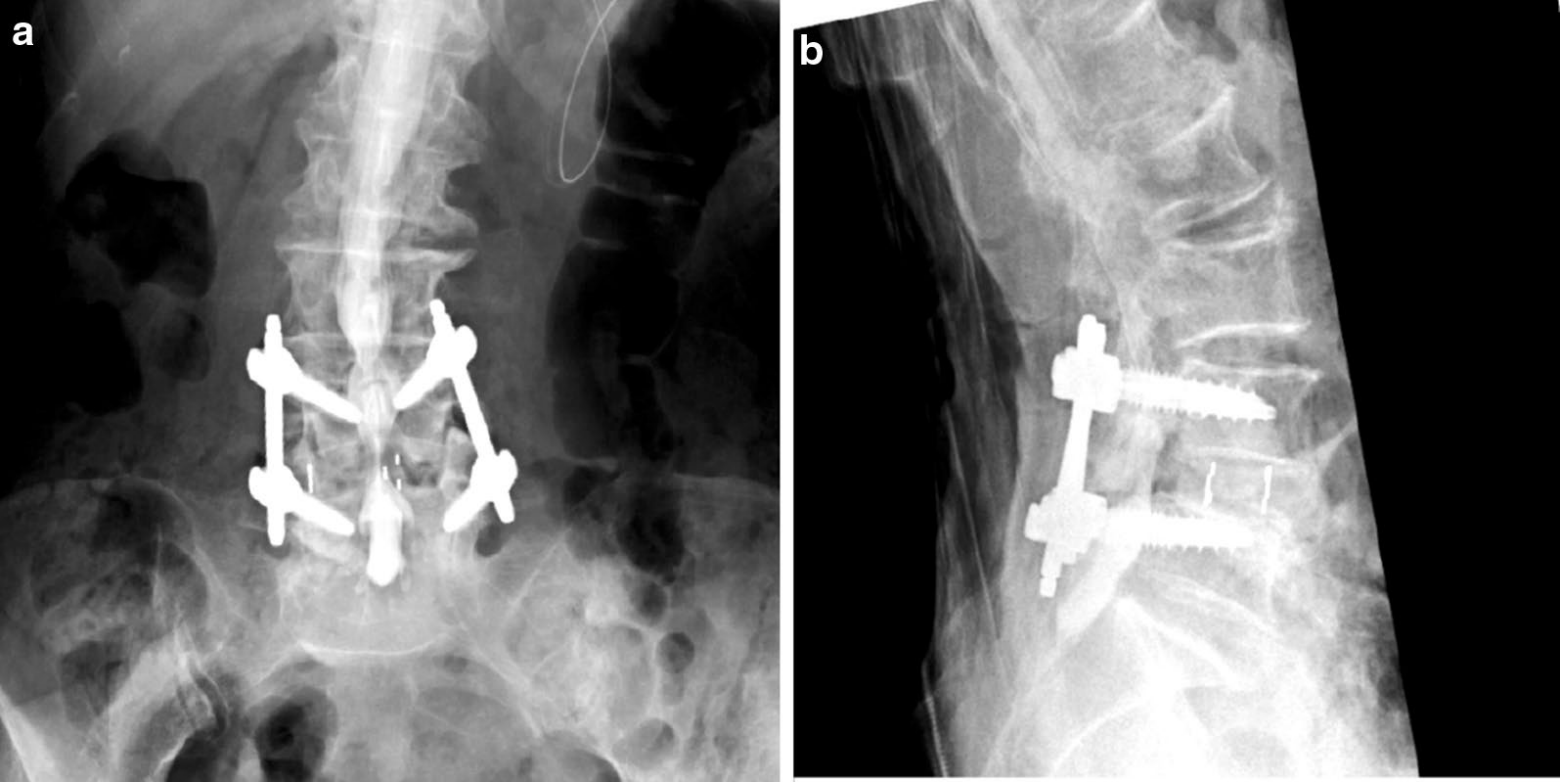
Postsurgical radiography. a Antero-posterior view. b Lateral view
